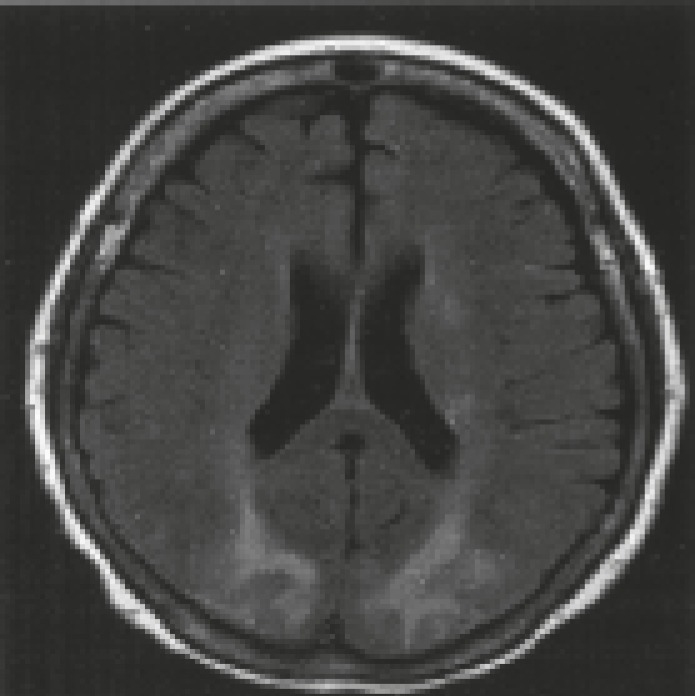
FLAIR MRI demonstrating bilateral zones of subcortical white matter hypersignal in the posterior frontal and occipital lobes
